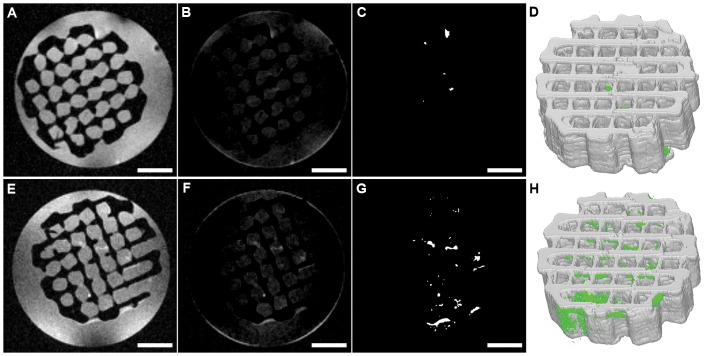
Image processing steps on MRI-derived image stacks in the absence (top, images A-D) and presence (bottom, images E-H) of hMSCs (donor 1, 5 weeks of culture). A and E represent the unprocessed MR images; B and F the images after background subtraction; C and G show the results of segmentation based on a single intensity threshold within a circular ROI, comprising scaffold and surrounding water within the MRI glass tubes; D and H are the obtained 3D mesh models, representing the tissue localization, amounts and density. Scale bars represent 2 mm.
